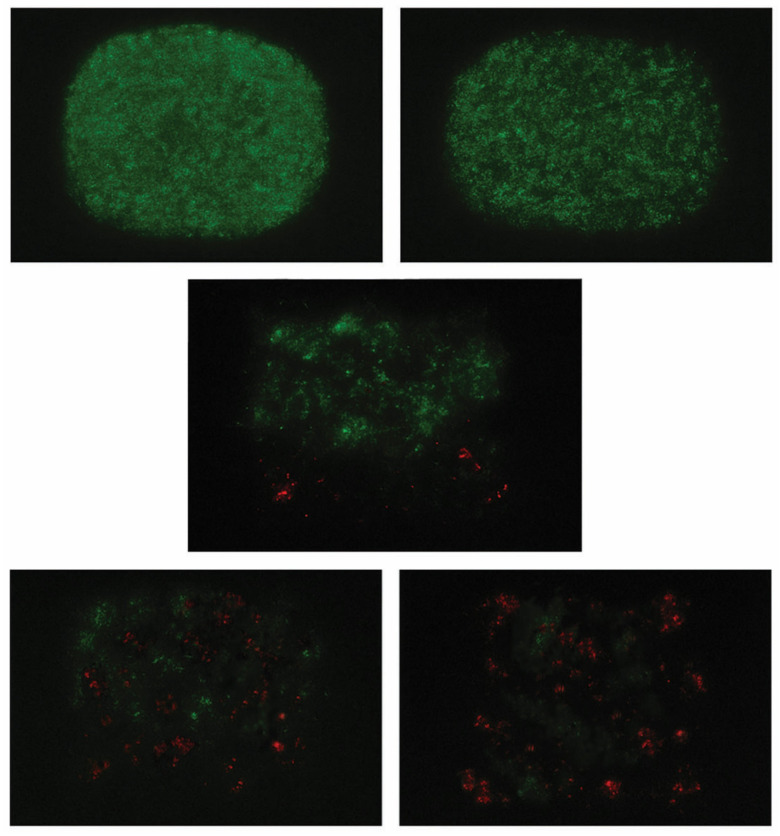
Representative CLSM images of bacterial biofilms from different treatment groups. The control group (top left corner) showed densely aggregated, rounded biofilm structures with homogeneous fluorescence intensity. For the saline control (top right corner), a similar fluorescence distribution but with a slightly lower packing density was observed. Ultrasonic debridement alone (middle bottom) disrupted biofilm organization, yielding patchy green fluorescence interspersed with localized red fluorescence. When combined with St. John’s extract (bottom left corner) and chlorhexidine (bottom right corner), this treatment induced sparse biofilm, with increased red fluorescence and scattered green.
